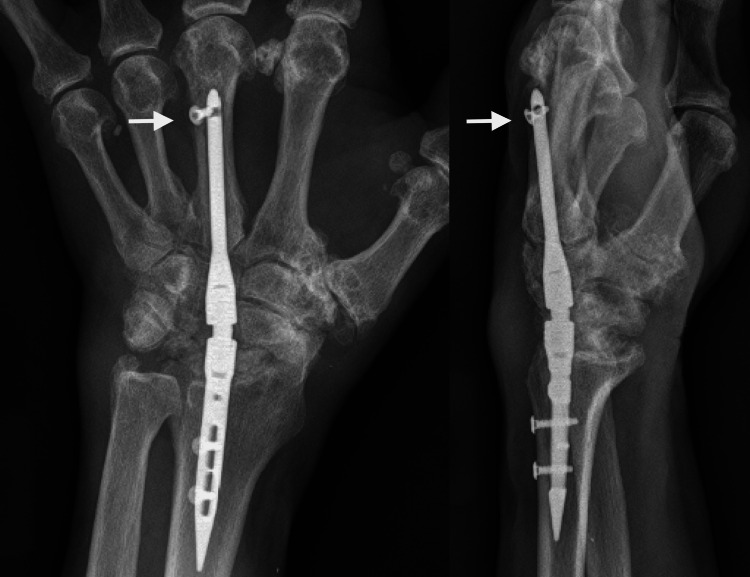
Follow-up radiographs of Case 3 Posteroanterior and lateral views at 3.5 months postop show that the distal unicortical screw backed out. The white arrows show the distal screw loosening and migration.
